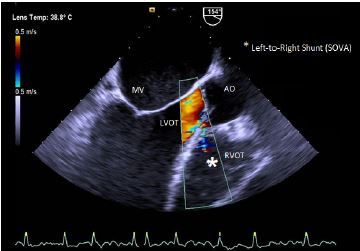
Tranesophageal echocardiogram demonstrating a ruptured sinus of valsalva aneurysm (star). *MV*, mitral valve; *Ao*, aorta; *RVOT*, right ventricle outflow tract; *LVOT*, Left ventricle outflow tract.
